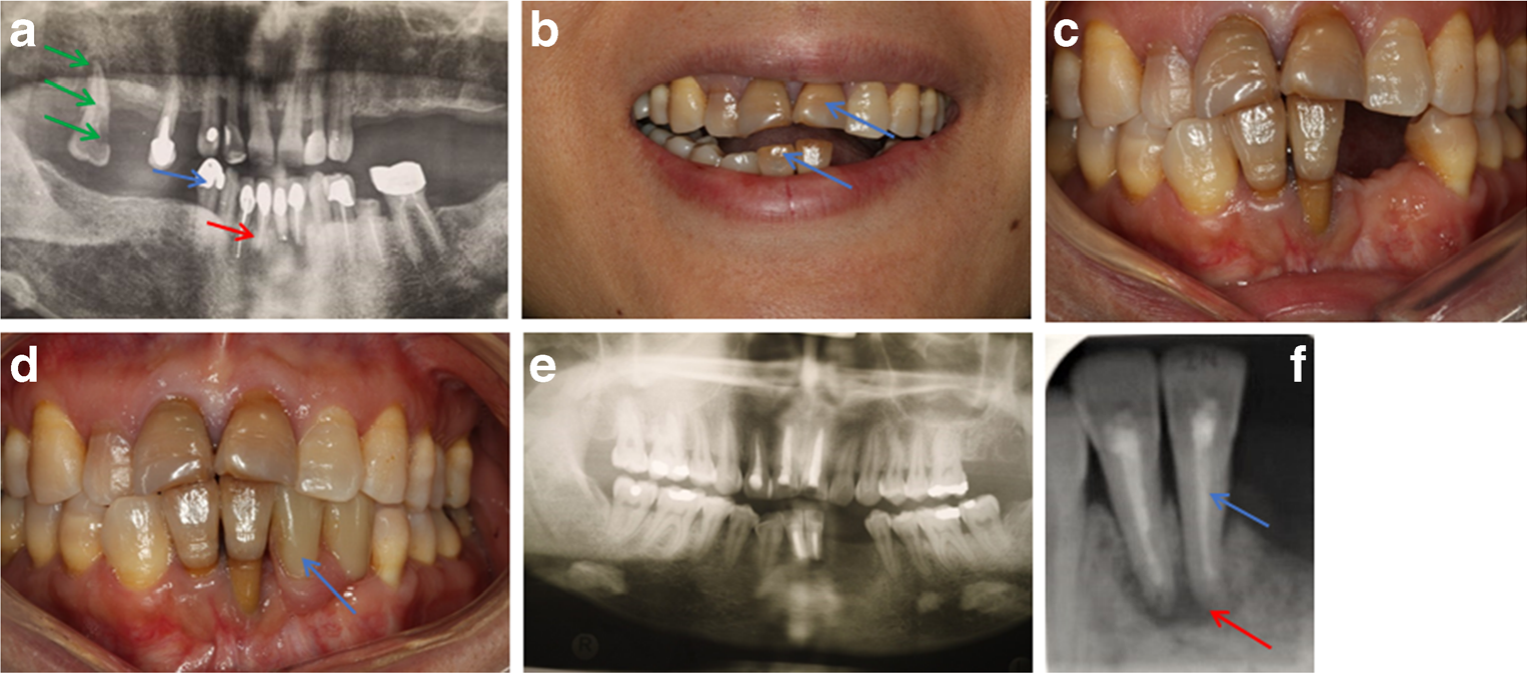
Dental complications of XLH. a Radiograph of a patient showing taurodont molars. Large pulp chambers are evident with an abscess on the upper right molar and large root canals (green arrows). Multiple teeth have been root filled (red arrow) and crowned (blue arrow). b Patient presenting with recurring abscesses associated with the lower incisor teeth. Note the discolouration (blue arrows) of the lower and upper incisor teeth (the 2 middle teeth). c Intraoral view showing the teeth; this patient had presented with unstable periodontal (gum) disease and caries (decay) which has now been treated and 2 teeth have been lost. d Patient wearing a lower denture (blue arrow) to replace the missing lower left incisors. e-f Dental pantomogram showing root fillings (blue arrow) in the upper right (UR) 2, 1 and upper left (UL) 1 and the lower right (LR) 1, 2 with wide canals and missing lower left (LL) 1, 2. The periapical view of the LR 1, 2 shows the apical infection and the unusual appearance of the bone (red arrow)
